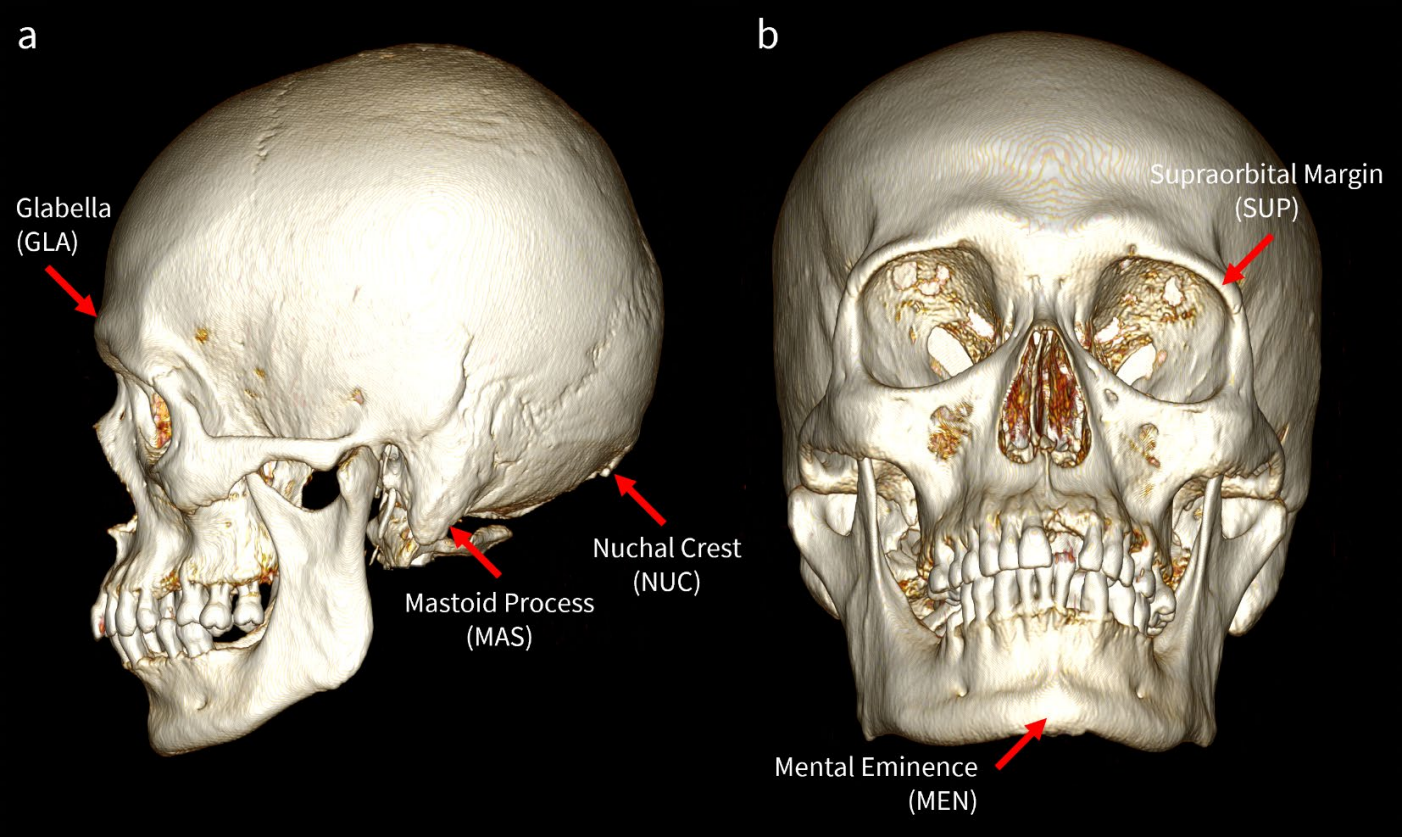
Volume-rendered CT scan visualised in OsiriX^®^, featuring the five cranial traits used in this study. (a) Left lateral view, with the glabella (GLA), mastoid process (MAS), and nuchal crest (NUC) indicated. (b) Inferio-anterior view, with the mental eminence (MEN) and supraorbital margin (SUP) indicated.
